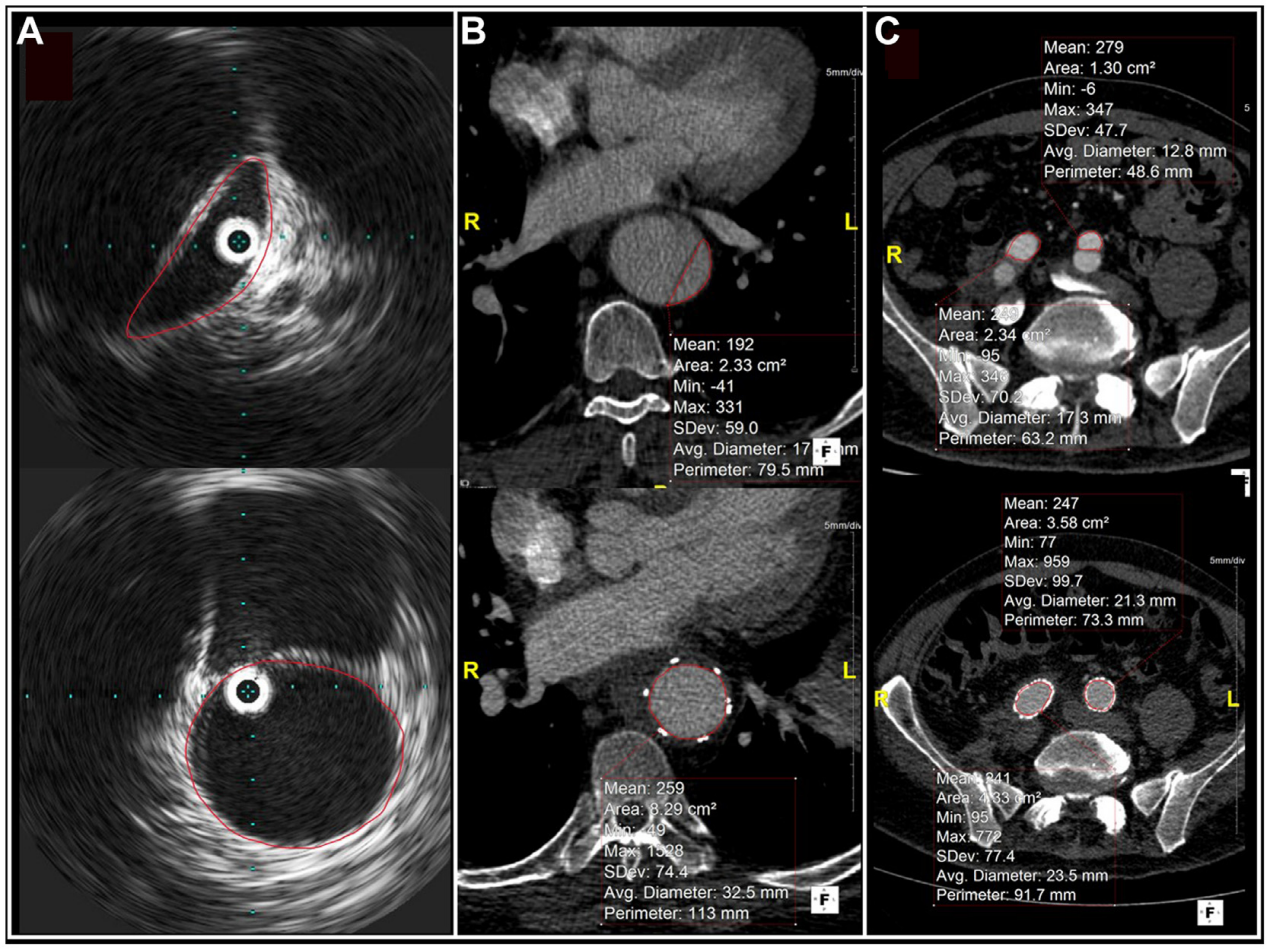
Representative comparative images from intravascular ultrasound (IVUS; A) and computed tomography angiography (CTA) of the aorta (B) and common iliac arteries (C) before and after transcatheter electrosurgical aortic septotomy (TEAS).
